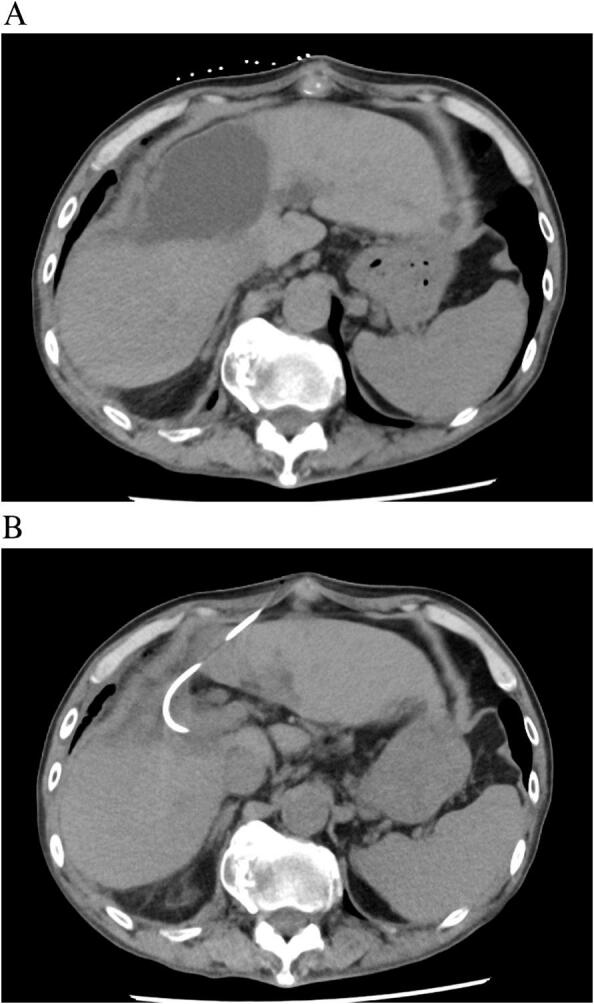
Enlarged fluid collection on the resected liver surface. CT on the 60th postoperative day showed enlargement of fluid collection on the resected liver surface (A). CT-guided percutaneous drainage was performed (B).
